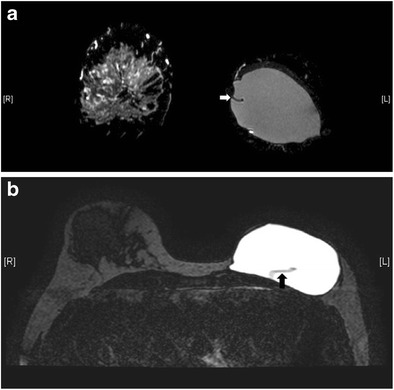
Radial folds. T2-weighted fat-saturated coronal (a) and silicone-only axial (b) MR images of the same patient with silicone gel-filled implants show a hypointense line running from the periphery, which is perpendicular to the normal intact left silicone gel-filled implant shell, suggestive of a radial fold (arrow)
